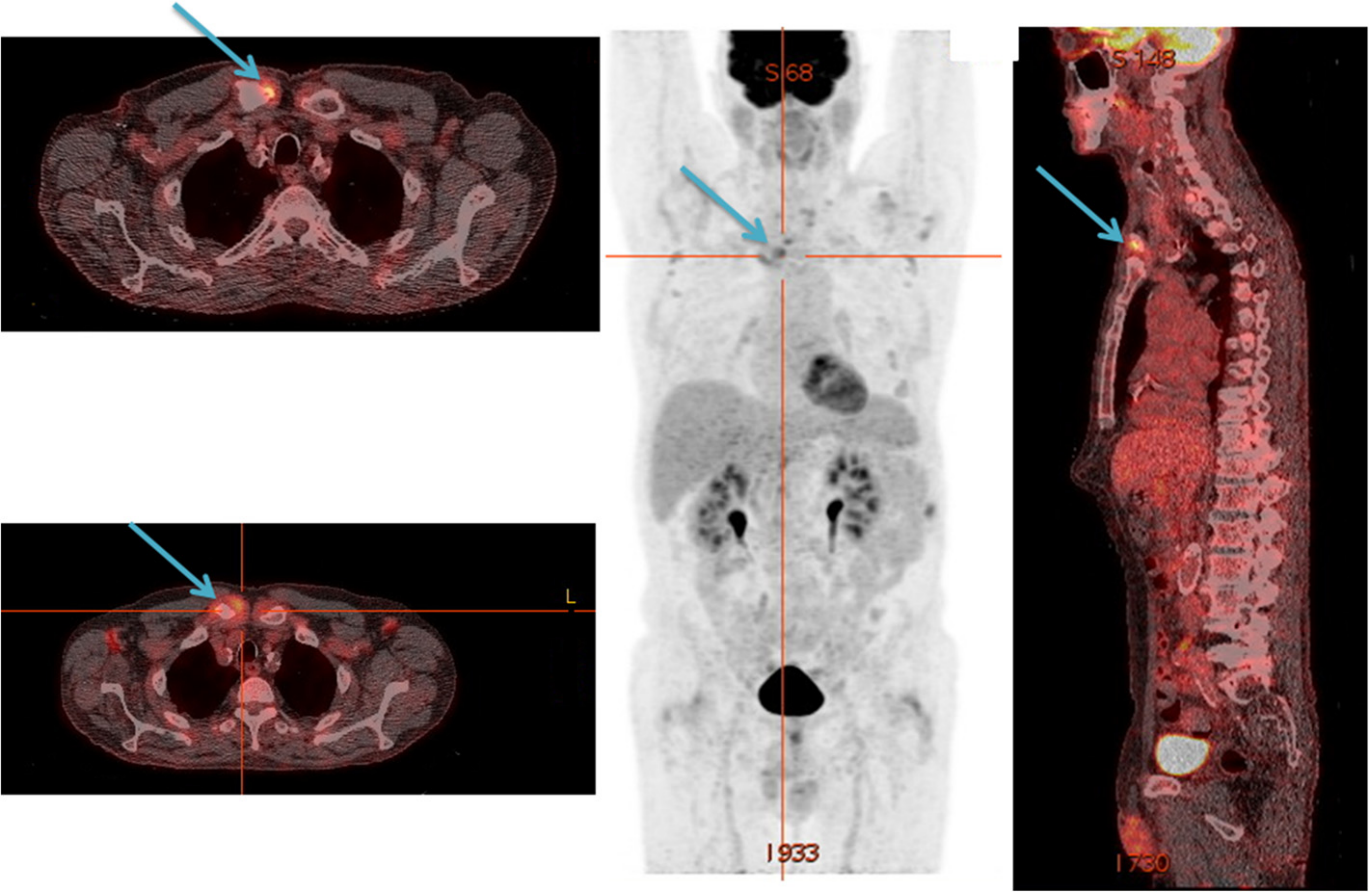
^18^F-fluorodeoxyglucose positron emission tomography computed tomography in a patient with *Coxiella burnetii* sternoclavicular joint infection. The high fluorodeoxyglucose uptake (*arrows*) is observed at the level of the sternoclavicular joint
